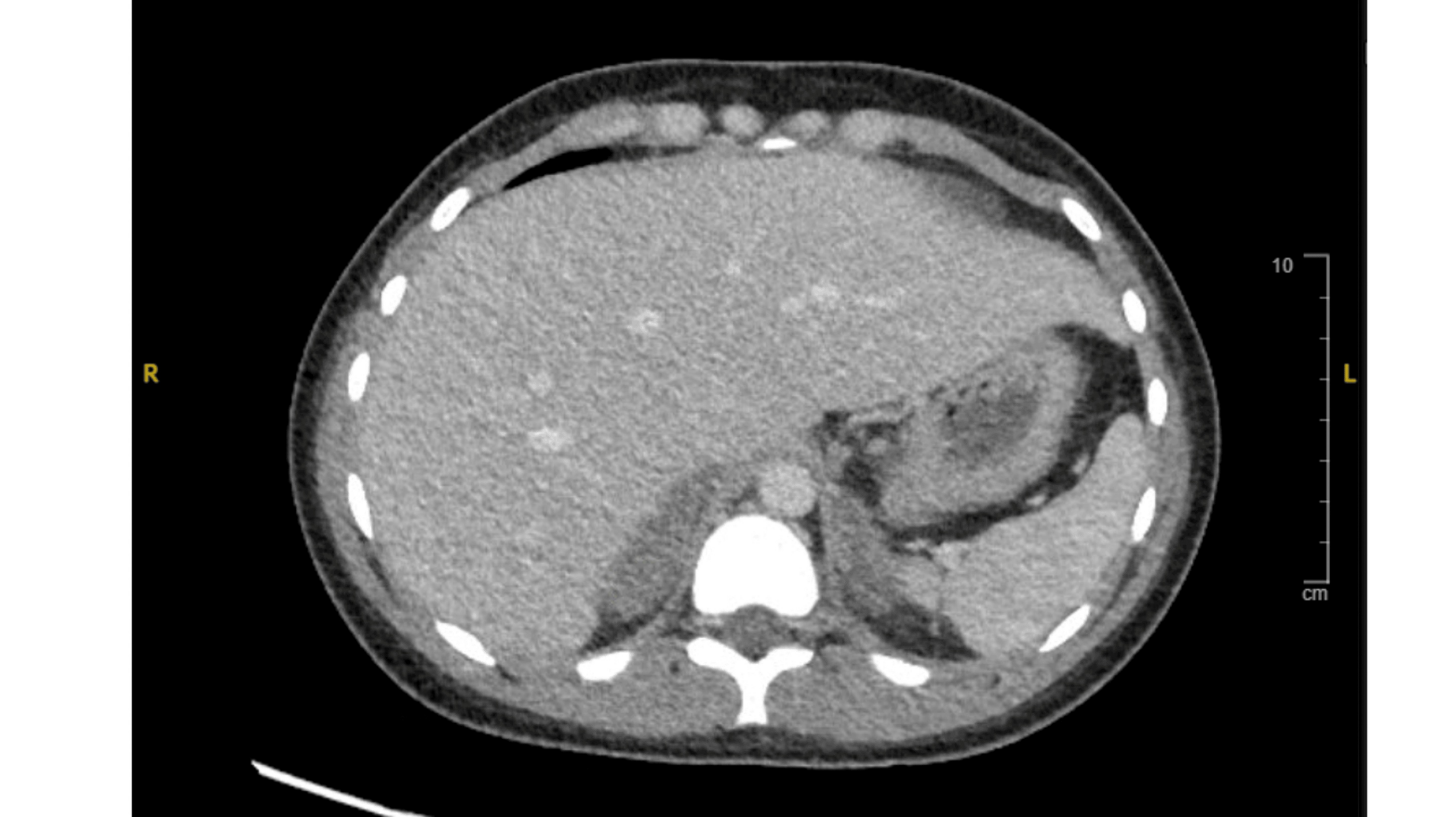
CT of the abdomen with and without contrast. There is marked bilateral adrenal gland thickening.
